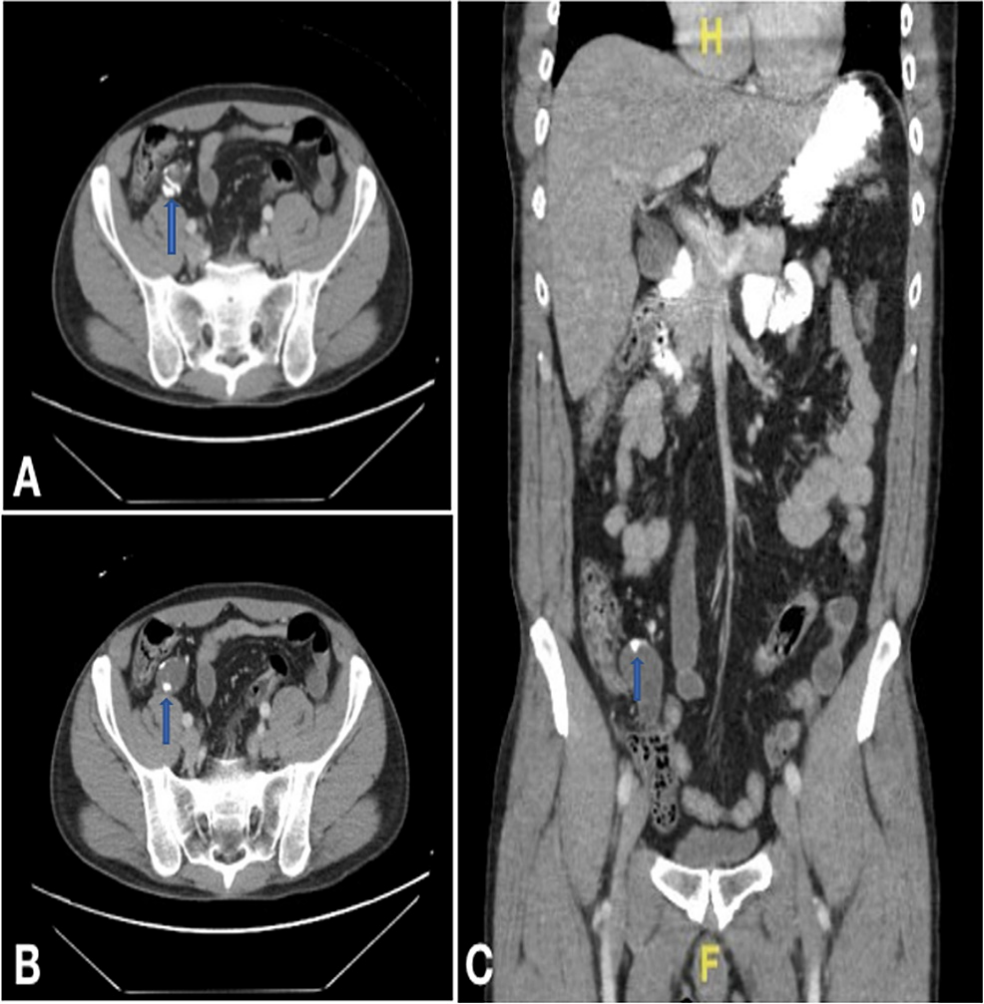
Admission CT of abdomen and pelvis A. Axial format. B. Axial format. C. Coronal format CT: computed tomography
